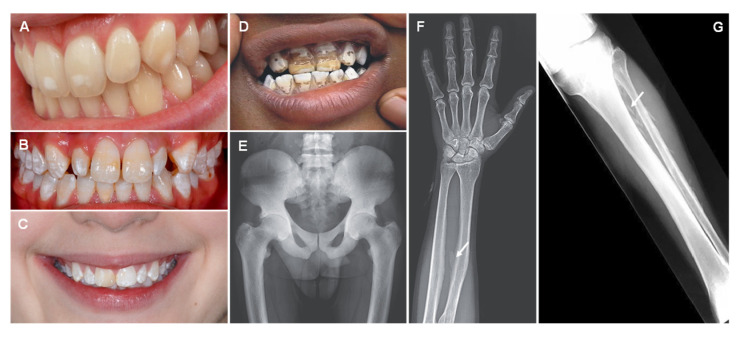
Dental fluorosis: mild (A), moderate (B,C), and severe (D). Pelvic radiography showing osteosclerosis (E). X-ray with arrows showing skeletal fluorosis evidenced by the diffuse bone condensation and interosseous membrane calcification of the forearm (F) and leg (G). Reprinted from (B)—[127], (D)—[128], (E,F)—[129], (G)—[126].
